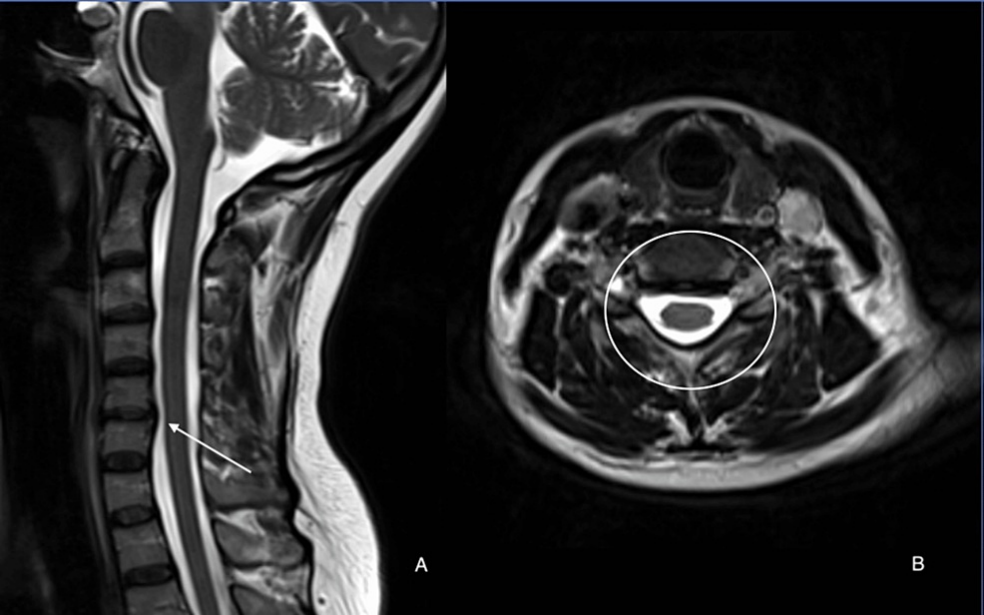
Repeat cervical MRI done 10 months after presentation showing regression of the previously documented cervical disc. MRI, magnetic resonance imaging
